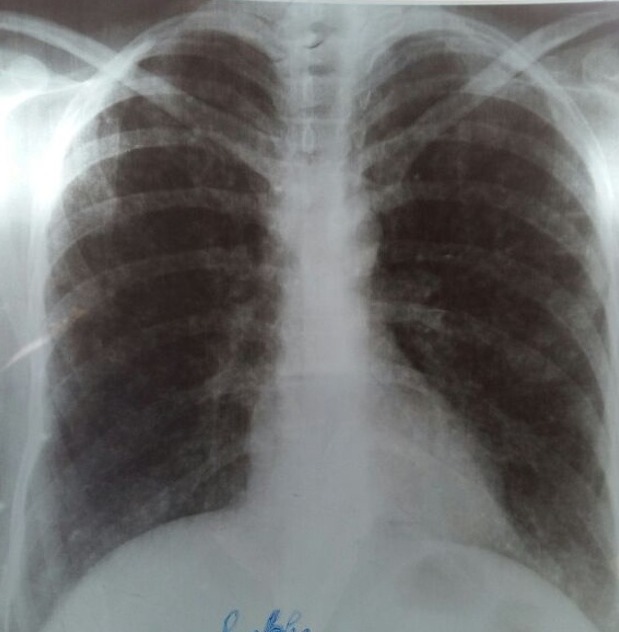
radiographie thoracique de face: opacités micronodulaires diffuses aux deux champs pulmonaire réalisant un aspect de Miliaire (1^ère^ observation)
